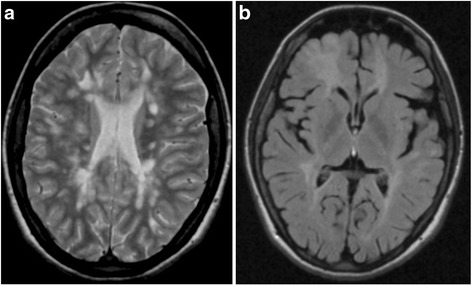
a Dual Fast SE, Dual Fast Spin-Echo, transverse (2011): Enlargement of the atypical hyperintensive tumefactive demyelinated lesion in the right frontal lobe. b FLAIR, Fluid -Attenuated Inversion Recovery, transverse (2011): Enlargement of the atypical tumefactive demyelinated lesion in the right frontal lobe
